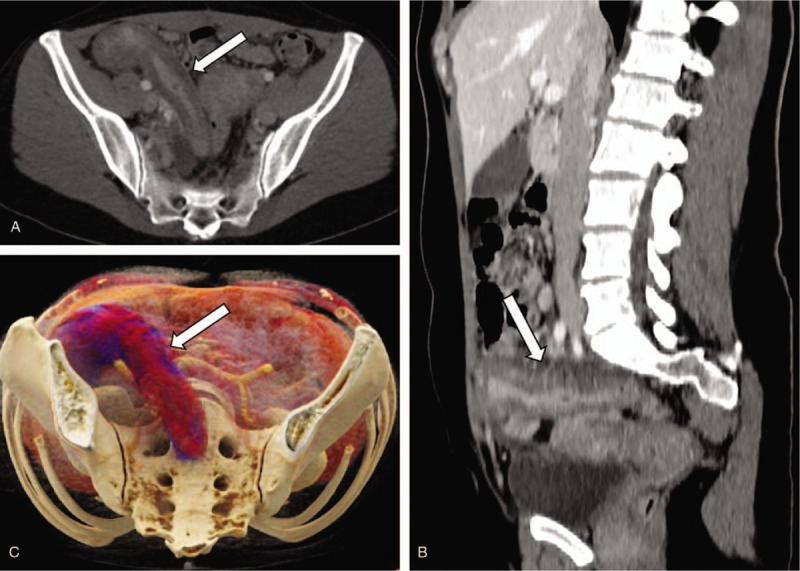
A 42-year-old woman, with IgG4-related appendiceal disease. Contrast-enhanced CT in axial plane (A), oblique sagittal plane (B), and 3D cinematic volume rendering reconstruction in axial plane (C) demonstrate the appendix with marked dilation, thickened walls, and mucosal hyperenhancement (arrows). CT = computed tomography, IgG4 = immunoglobulin G4.
